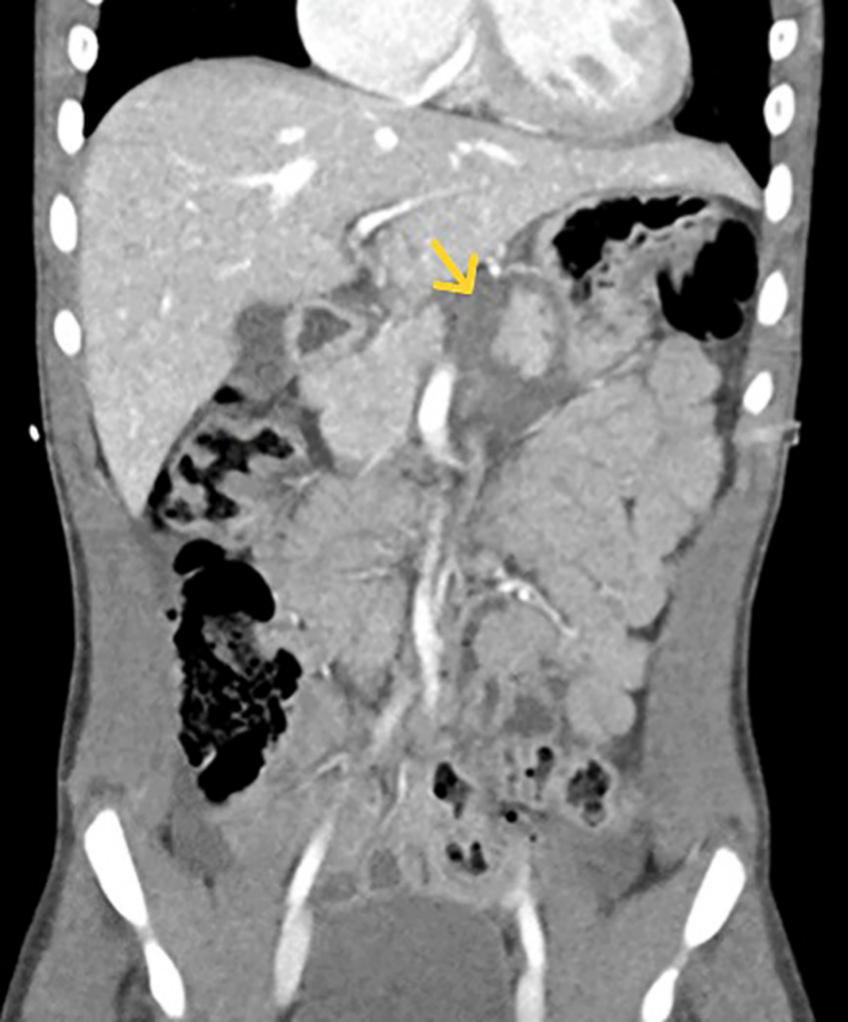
Computed tomography (CT) abdomen demonstrating a pancreatic pseudocyst (indicated by the yellow arrow).
